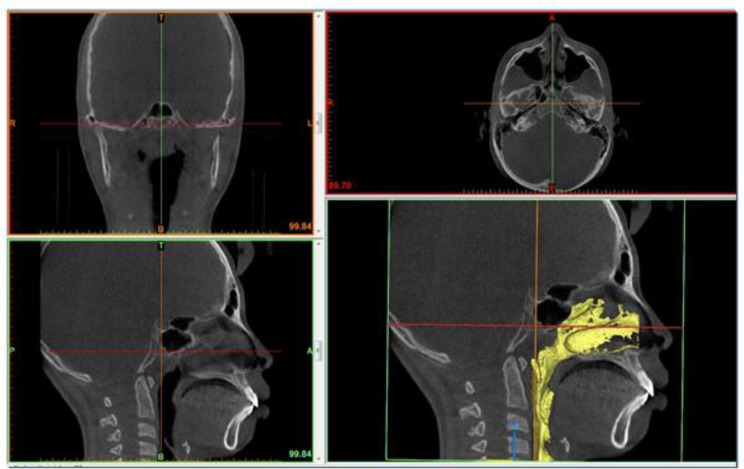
Clinical radiograph of case using Myobrace (T4A^TM^): Before treatment CBCT
